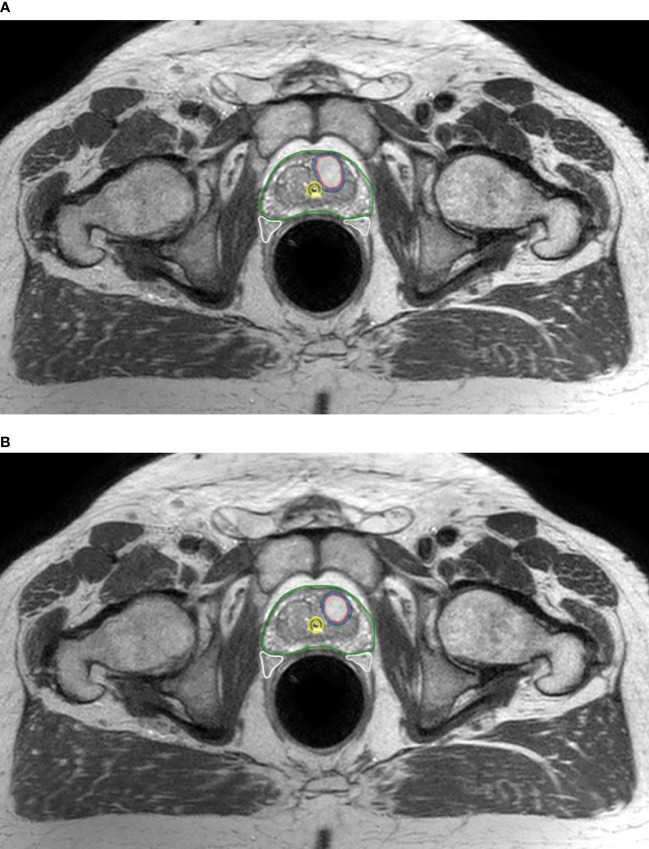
^68^PSMA-detected relapse in a patient who received 5-fraction SABR. (A) Fused ^68^PSMA-PET/CT and planning MRI show pretreatment location of ^68^PSMA-detected dominant intraprostatic lesion (DIL) encompassed within the CTV and receiving the full 45 Gy prescription dose; (B) fused ^68^PSMA-PET/CT acquired at the occurrence of biochemical relapse with the planning MRI scan shows persistence/recurrence of the DIL at the initial site.
